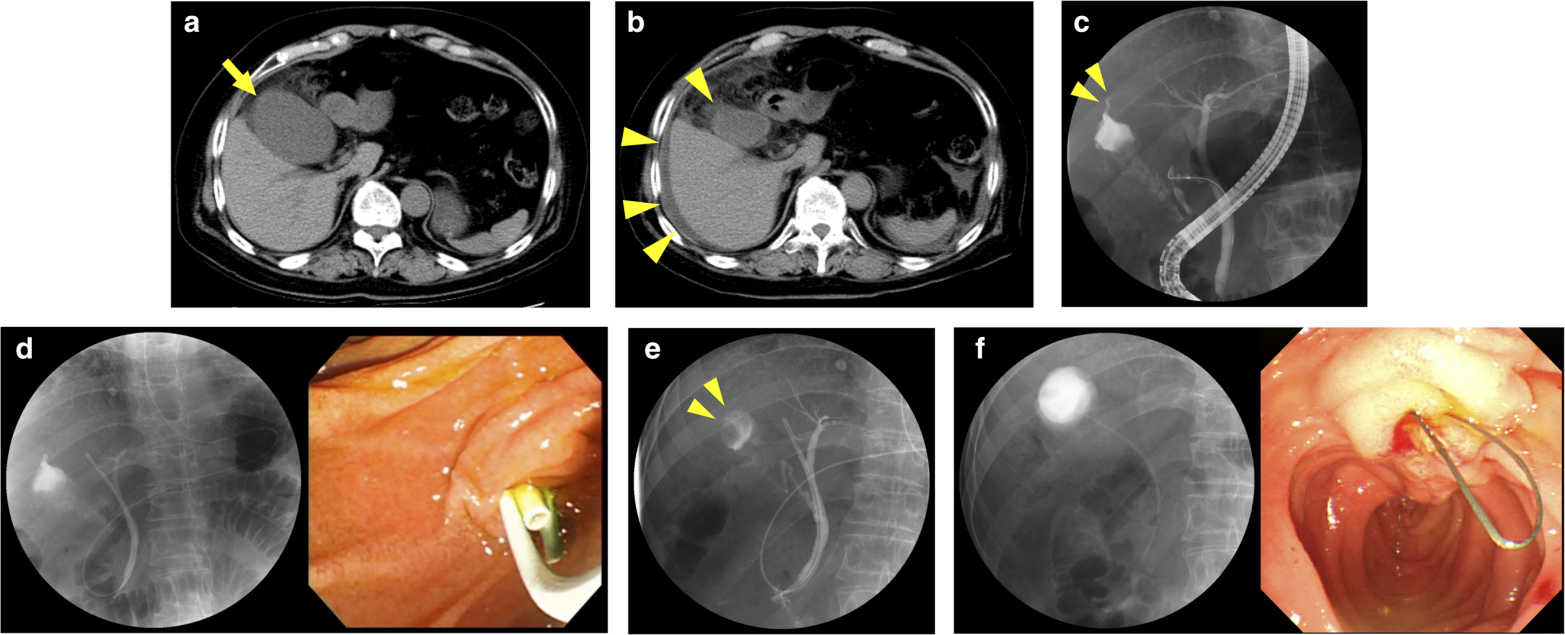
(a) Computed tomography (CT) revealed the enlarged gallbladder with pericholecystic inflammation (arrow). (b) CT showed the appearance of ascites with the reduction of gallbladder swelling (arrowhead). (c) Endoscopic retrograde cholangiopancreatography demonstrated leakage of the contrast medium from the wall of the gallbladder fundus (arrowhead). (d) A 7‐Fr plastic stent (PS) was placed in the right intrahepatic bile duct, and a 5‐Fr endoscopic nasobiliary drainage catheter was placed in the left intrahepatic bile duct. A 5‐Fr PS was placed in the main pancreatic duct. (e) Fluoroscopic image revealed no leakage from the gallbladder into the abdominal cavity (arrowhead). (f) A 5‐Fr single pigtail stent with thread (Biliary stent with thread; CX‐T stent; Gadelius Medical, Tokyo, Japan) was placed in the gallbladder.
